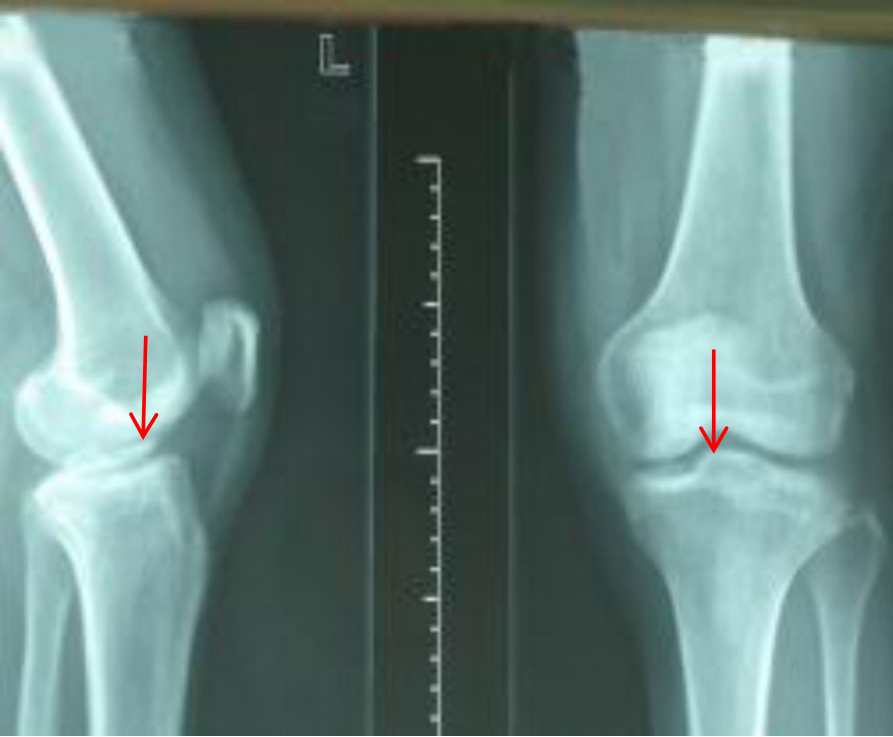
X-ray image showing an osteochondral fragment on the tibial plateau that may be confused for an avulsion fracture of the tibial insertion of the anterior cruciate ligament (*red arrow*)
